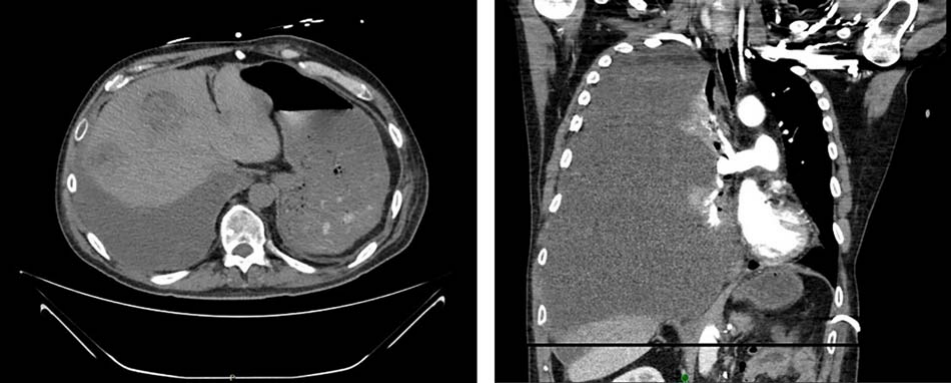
CT demonstrates growth of effusion into the entirety of the right hemithorax with mass effect shifting all thoracic contents to the left (left); later cytology ruled out malignant effusion; substantial increase in liver metastatic lesions can also be seen when compared to previous images from 2 months prior.
